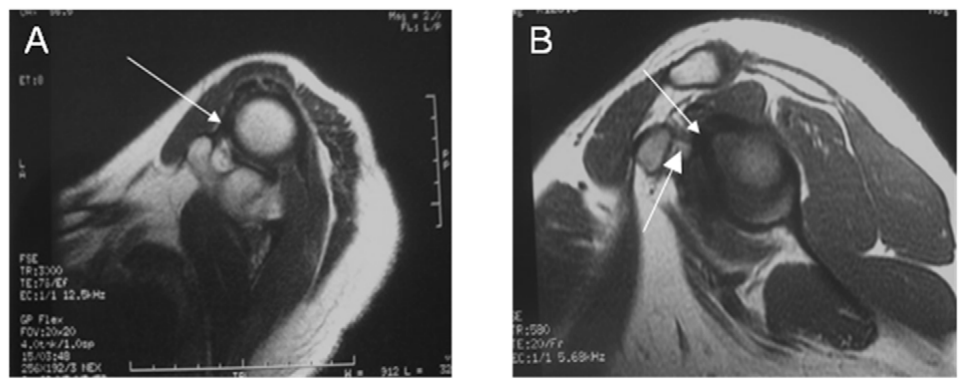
MR image shows thickened and slightly twisted CHL (arrow) in a 52-year-old patient with AC in axial plane T1WI (A) and in sagittal oblique plane (B).
